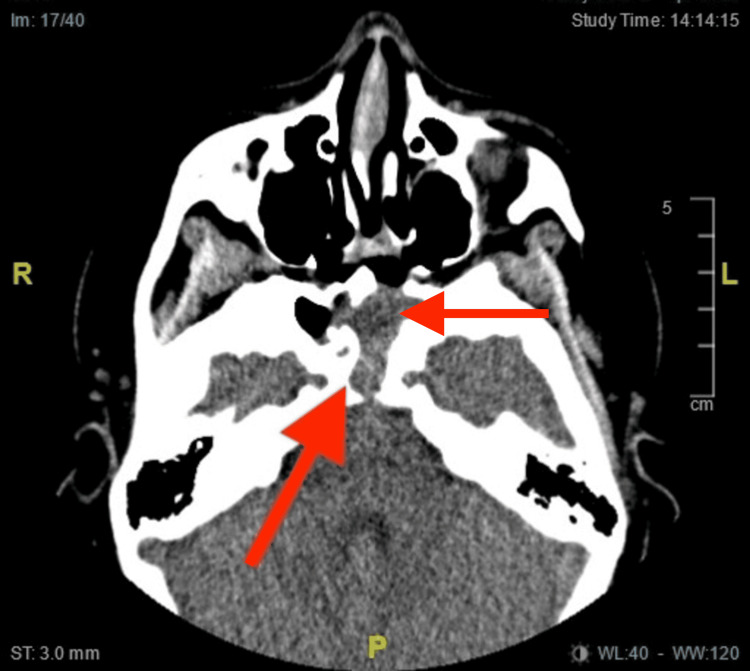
In the CT scan of the paranasal sinuses, postsurgical changes due to sphenoidotomy were identified with enlargement of the sphenoid ostium. Hypodensity of soft tissue was noted, with partial occupation of the sphenoid sinus extending to the sphenoid ostium. This was associated with bone erosion and destruction of the clivus, extending to the posterior wall of the clivus (red arrow).
